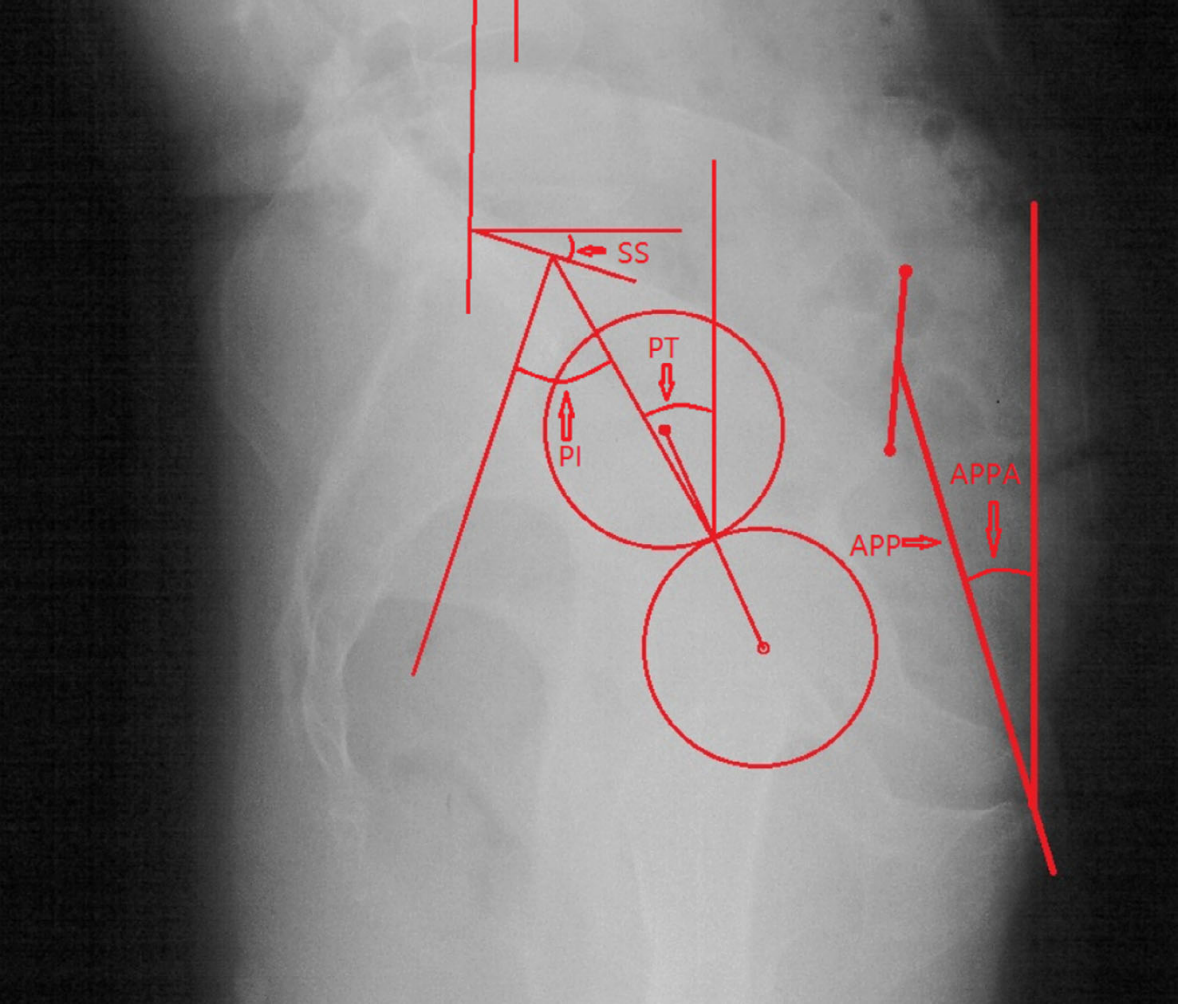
The anterior pelvic plane (APP) was the plane formed by both anterior superior iliac spines and the pubic symphysis. APPA was defined as the angle between the line connecting the midpoint of both anterior superior iliac spines to the pubic symphysis and the vertical line of the lateral radiograph of the pelvis in the standing position. Pelvic incidence (PI) is the angle formed by the line connecting the midpoint of the bilateral femoral head center point to the center point of the sacral endplate and the line perpendicular to the sacral endplate. Pelvic tilt (PT) is the angle between the line connecting the midpoint of the bilateral femoral head center point to the center point of the sacral endplate and the vertical line; the sacral slope (SS) is the angle between the superior plate of S_1_ and the horizontal line.
